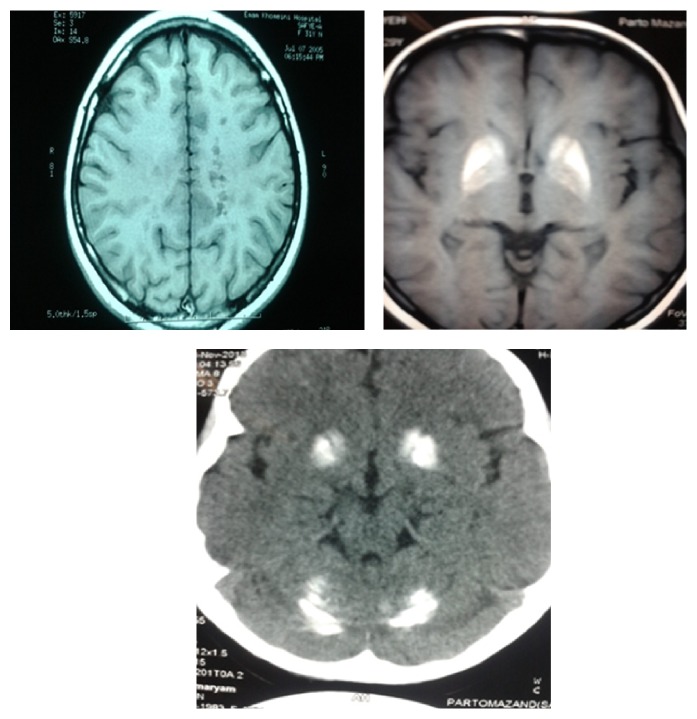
T1 MRI: black hole and basal ganglia calcification at upper side. CT scan: BG and cerebellar calcification at lower side.
